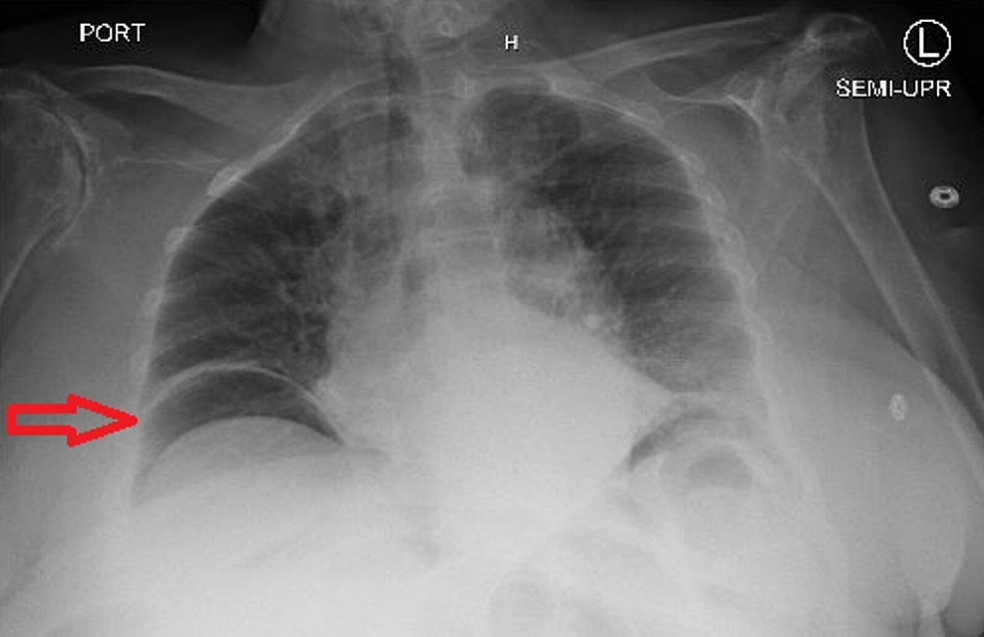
CXR showing air under diaphragm’s right dome (arrow) CXR: chest X-ray
